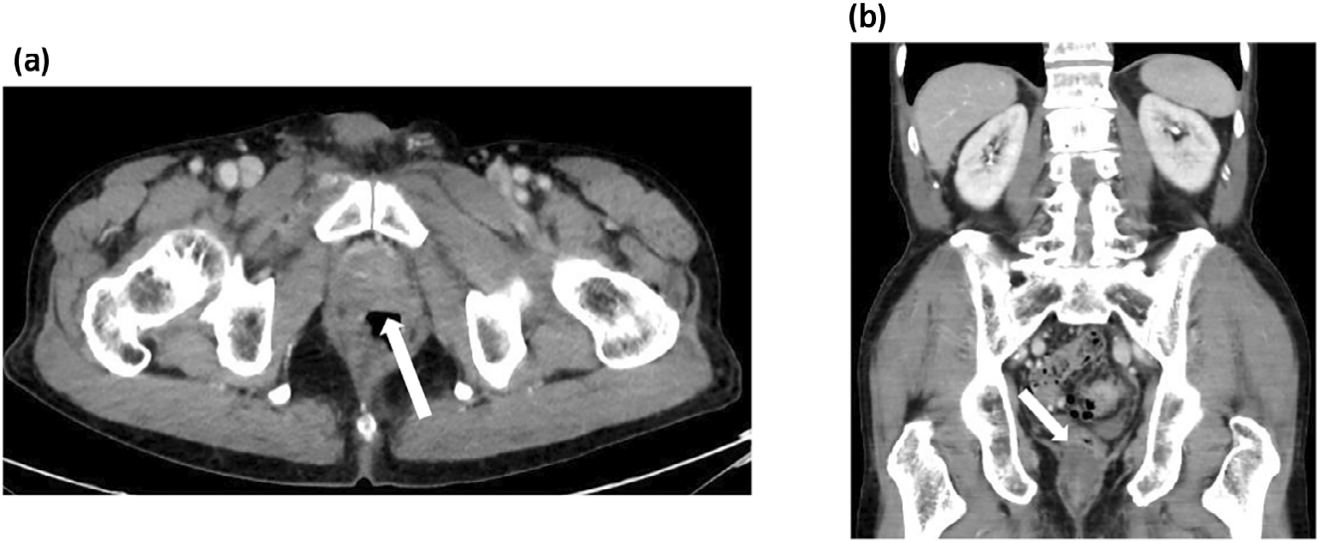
CT images after completion of treatment with combined antitubercular drugs. (a, b) The tuberculous prostatic abscess had almost completely disappeared after completion of the treatment with combined antitubercular drugs (white arrows; a, axial view; b, sagittal view).
